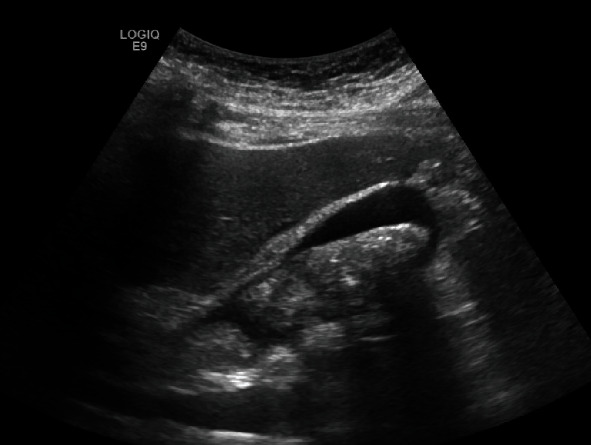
Abdominal ultrasound shows sludge in the gallbladder, gallbladder wall thickening, and pericolecystic fluid.
